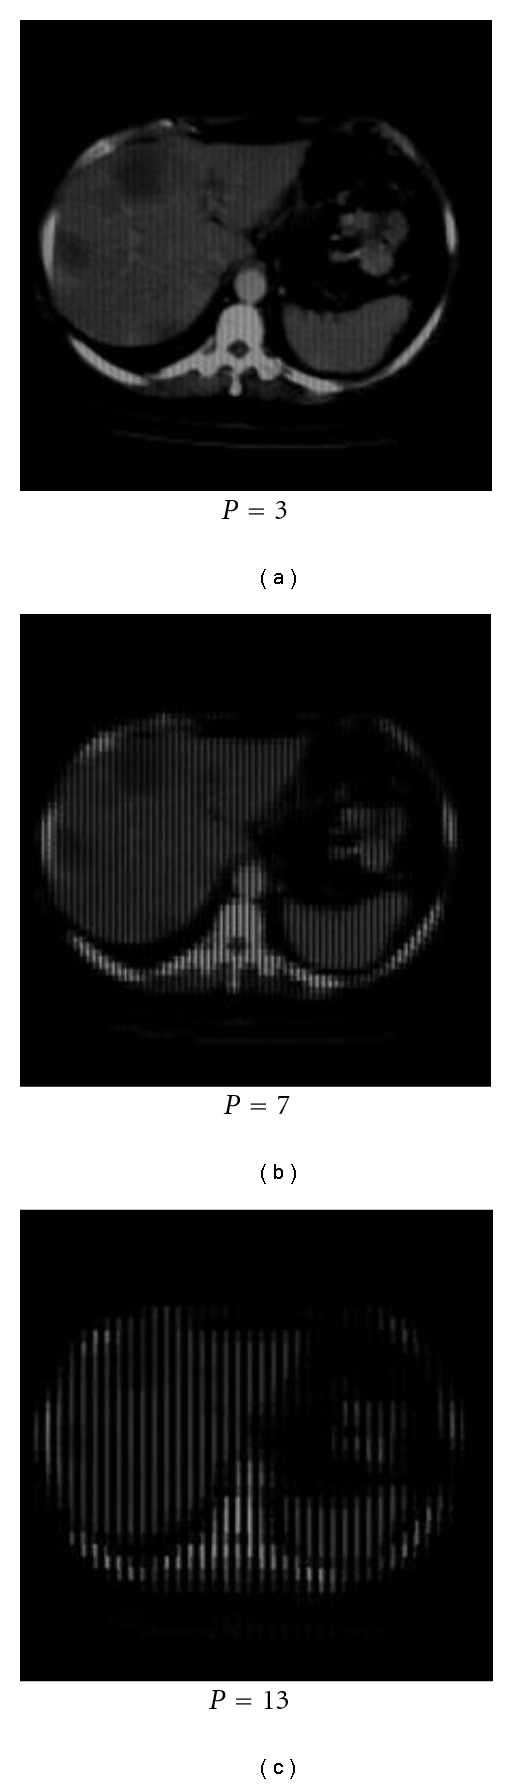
Ridgelet transform for real CT images at block sizes (3, 7, and 13).
